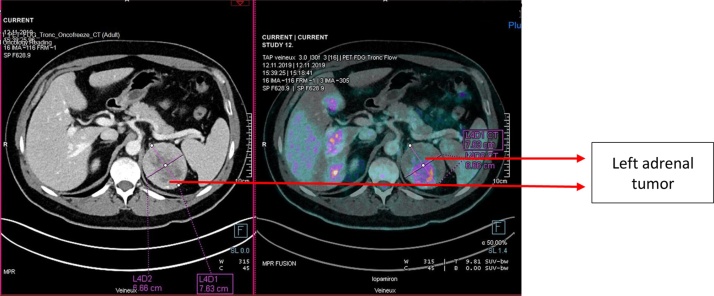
^18^FDG-PET-CT combined with contrast CT-scan showing the hypermetabolic left adrenal tumor, without distant metastases.
